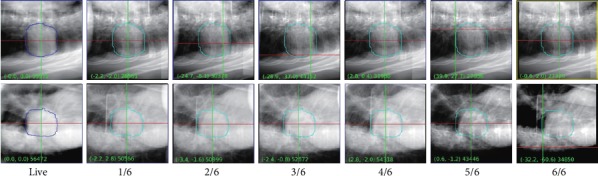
Comparing pixel density differences among real-time X-ray images of a 6-fraction case. Note: image guidance of a 6-Fractions patient. In the figure, live is the DRR image reconstructed from the CT localization image, and each sequence number is arranged as the real-time X-ray image taken in daily intervals.
